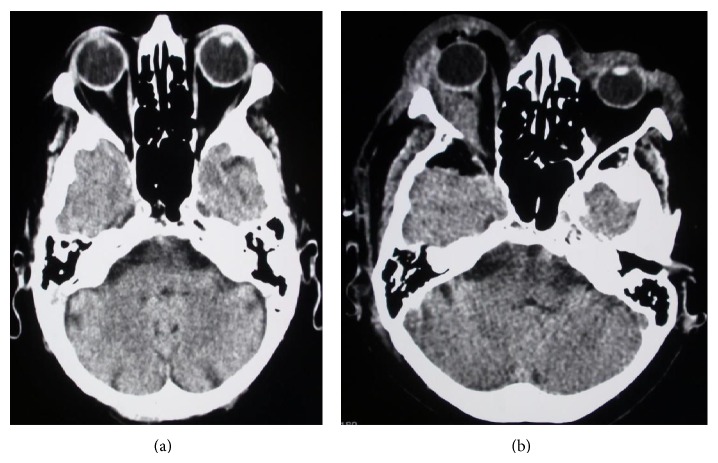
CT, pre- and postoperative: (a) Preoperative CT: intraorbital mass (thick arrow) and partially removed bone of the orbital roof (thin arrow) are seen. (b) Postoperative CT: the intraorbital mass has been removed and the exophthalmos is less clearly evident in comparison to preoperative images.
